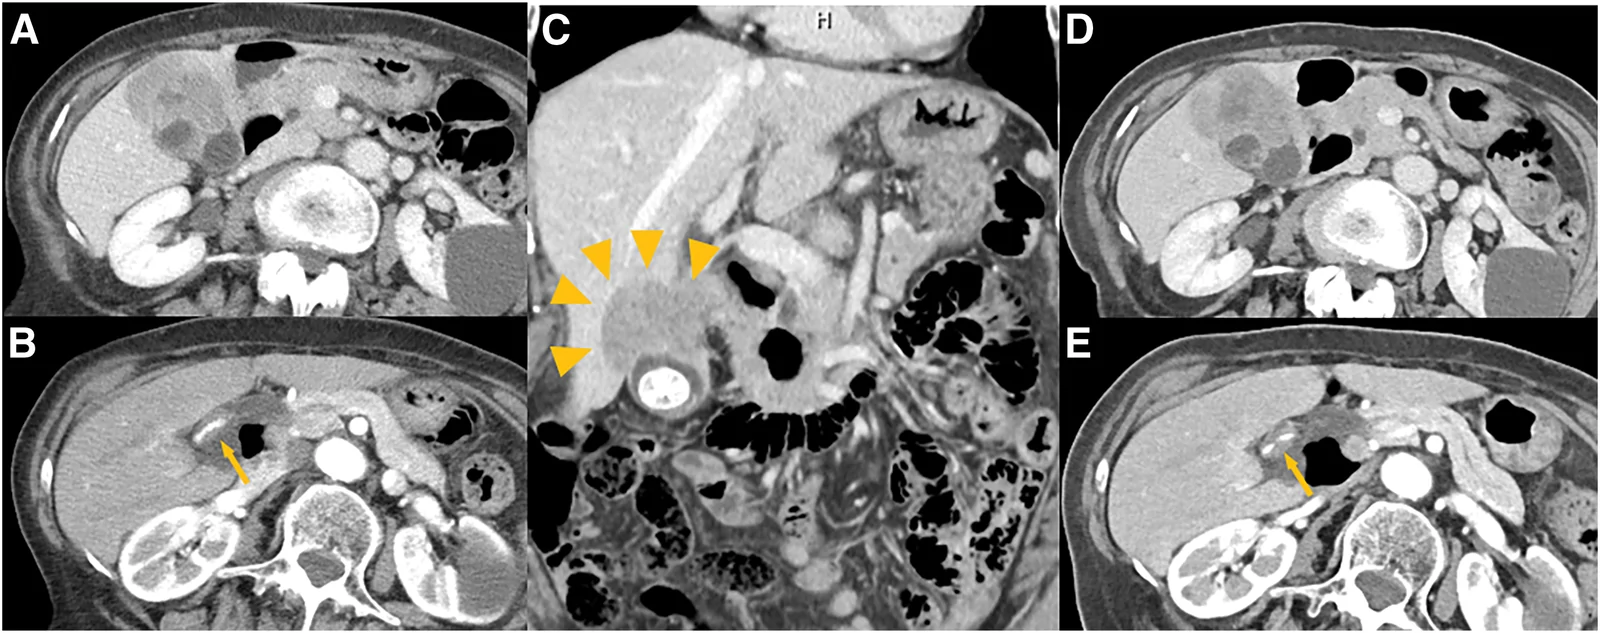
(A–C) Contrast-enhanced abdominal CT demonstrated thickening of the gallbladder fundus wall. The RHA appeared to be encased by enlarged hilar lymph nodes (arrow). The gallbladder tumor had invaded the adjacent liver parenchyma (arrowheds). (D, E) After 2 courses of GCD therapy, CT revealed that the primary lesion remained stable and that the RHA encasement had resolved (arrow). GCD, gemcitabine, cisplatin, and durvalumab; RHA, right hepatic artery
